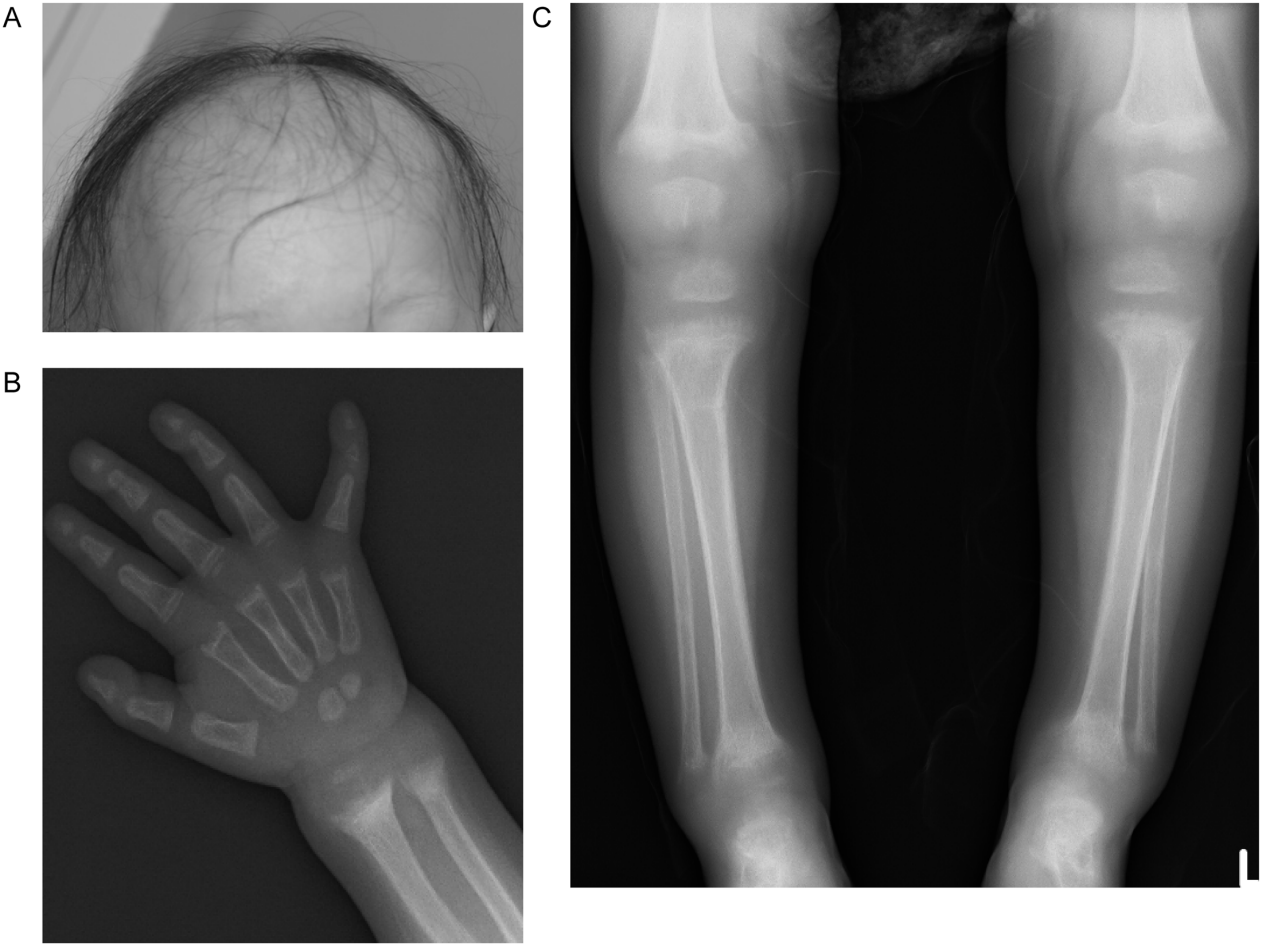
Alopecia and Rickets in the Proband at Presentation. (A) Alopecia. (B,C) A bone roentgenogram of the arm (B) and legs (C) showing cupping, fraying, and flaring at the end of the long bones.
